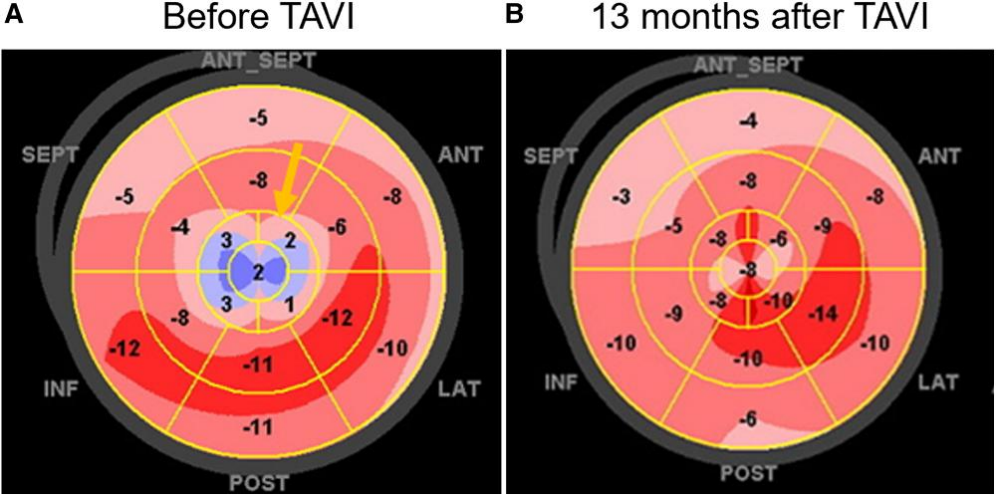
Global longitudinal strain as calculated by transthoracic echocardiogram demonstrating: (*A*) before TAVI and tafamidis administration. Especially, a significant wall motion abnormality was noted in the apical region (arrow). (*B*) Thirteen months after TAVI and tafamidis administration. Wall motion improved at the apex, and apical sparing was not observed.
